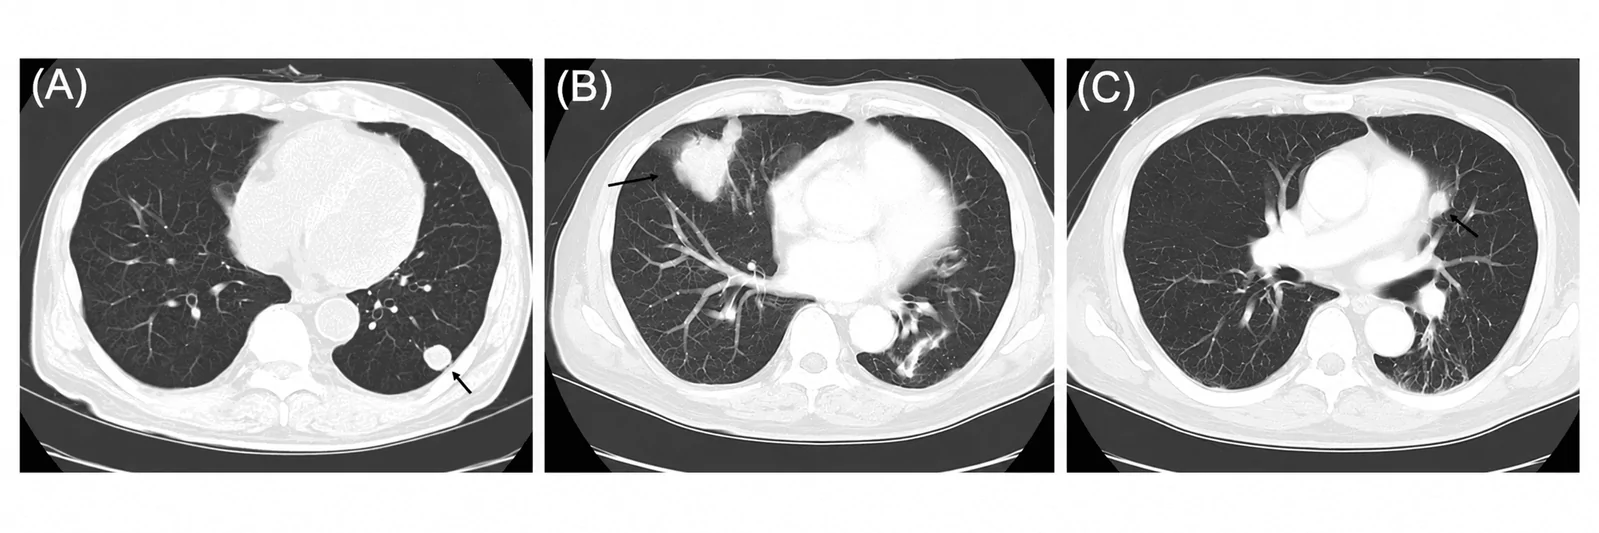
Chest CT Scans Two Months Prior to and at the Time of Radiation Oncology Consultation Chest CT images obtained two months before and at the radiation oncology consultation. (A) CT image obtained two months before consultation shows a left lower-lobe nodule (arrow), which was surgically resected and confirmed as a metastasis from hepatocellular carcinoma (HCC). (B, C) CT images at consultation show new nodules in the right middle lobe (B) and the lingula of the left lung (C) (arrows).
